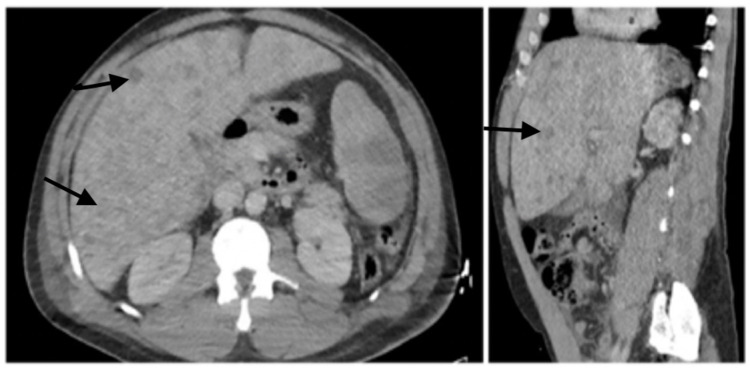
CT abdomen/pelvis scan with the presence of multiple hypodense liver lesions.
